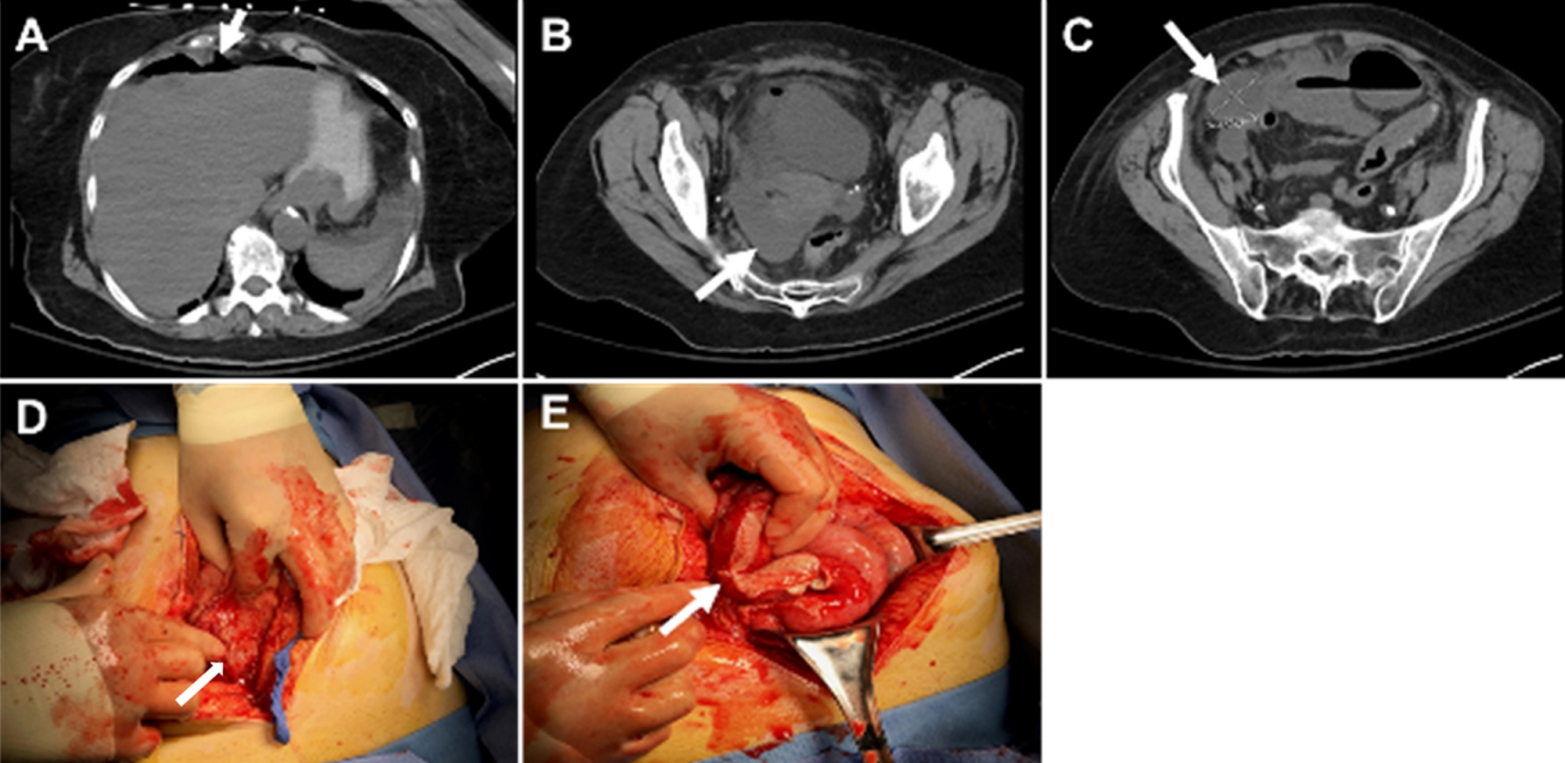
Perforated appendicitis with delayed presentation and septic abdomen, grade 2B open abdomen A. Pneumoperitoneum identified in the patient’s abdomen (arrow); B. Diffuse peritoneal fluid identified in abdominal CT scan (arrow); C. Diffuse peritoneal fluid identified in abdominal CT scan (arrow); D. Diffuse inflammation of bowel (arrow); E. Fibrin deposition involving the large and small bowel (arrow)
